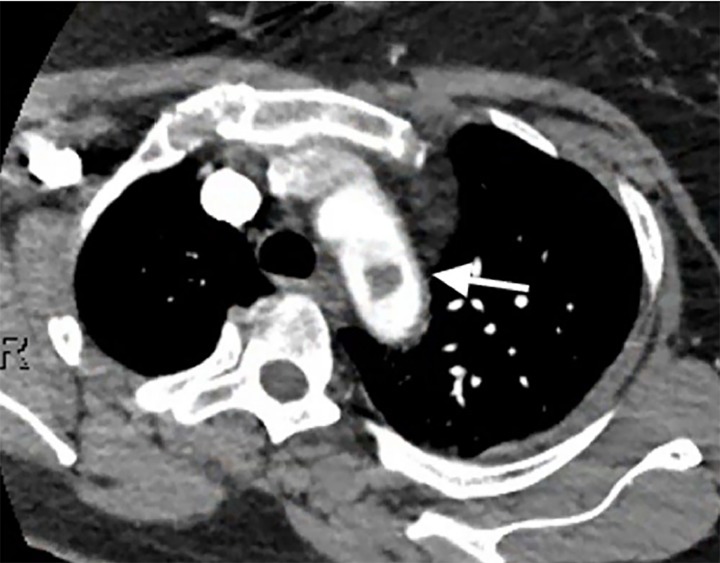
Computed tomography showing thrombus in the aortic arch (arrow).
